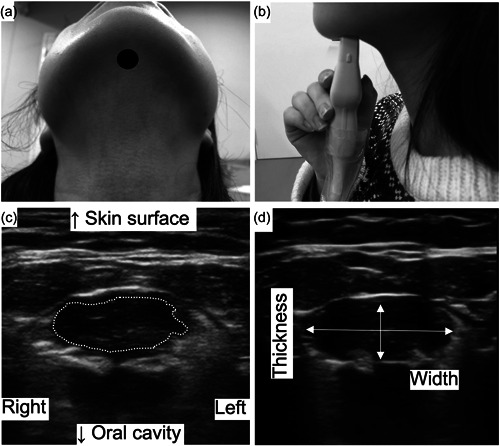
The ultrasound analysis of the geniohyoid muscle. (a) We assessed the distance between the mandible and the hyoid bone. We marked a position one‐third from the mandible as the measurement point. (b) We located a probe at the point in the coronal plane. (c) The white dotted line is the geniohyoid muscle. We positioned the probe at the marked point, traced the edge of the geniohyoid muscle, and measured the cross‐sectional area. (d) We measured the width and thickness of the geniohyoid muscle, as shown in (d). The horizontal arrow shows width, and the vertical arrow shows thickness. To avoid readers' confusion, we divided images for measurement, one for the area (c) and another for length (d).
